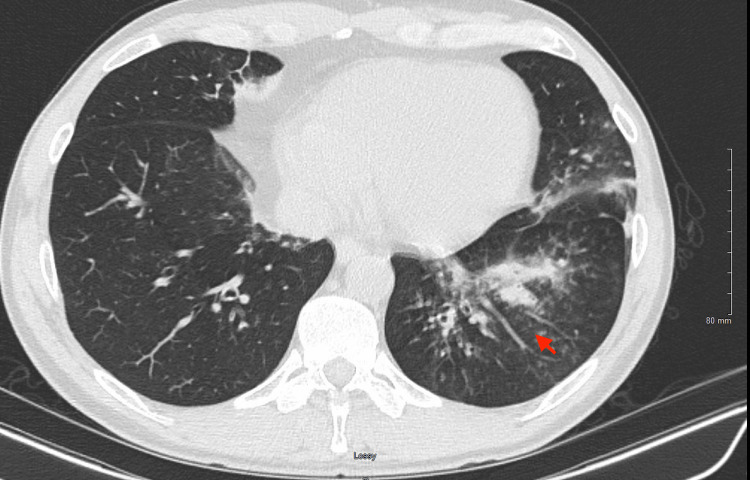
Chest CT prior to treatment initiation showing ground-glass opacity A chest CT prior to treatment initiation shows consolidation in the left lower lobe (red arrow) with associated peribronchial thickening.
